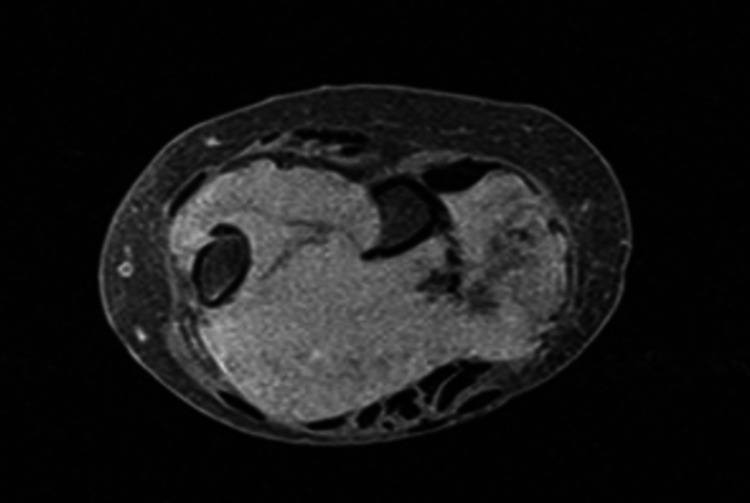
Axial view of the left wrist (MRI; T2W image). The lesion is round and well-circumscribed with an apparent enhancement of the periphery. MRI: magnetic resonance imaging; T2W: T2-weighted
